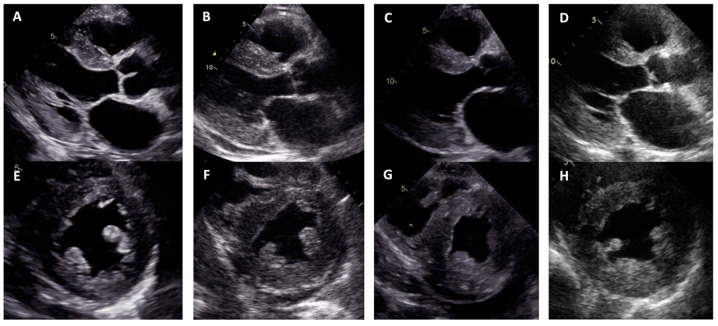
Concentric left ventricular hypertrophy in transthoracic echocardiography views: (A–D) parasternal long axis; (E–H) parasternal short axis; (A,E) proband, family 1; (B,F) proband, family 2; (C,G) proband, family 3; (D,H) proband, family 4.
